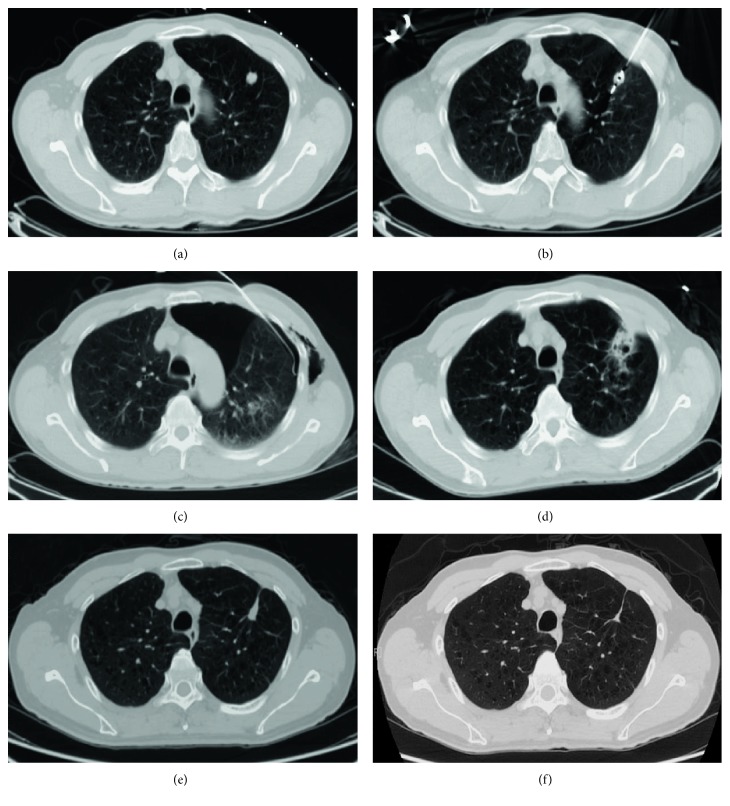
A 62-year-old man with lung metastases from colorectal carcinoma in the left upper lobe with a diameter of 1.5 cm (a). Ablation was performed after accurate insertion of MWA probe into the focus of tumor (b). The immediate postoperative CT scan showed pneumothorax (lung compressed approximately 40%), and thoracic cavity closed drainage was conducted immediately (c). The lesion enlarged slightly with ill-defined margin and air-filled cavity on one month follow-up (d). During 3 (e) and 6 (f) months follow-up, the lesion was shrunk gradually and replaced by fibrous tissue with no evidence of tumor remnants.
